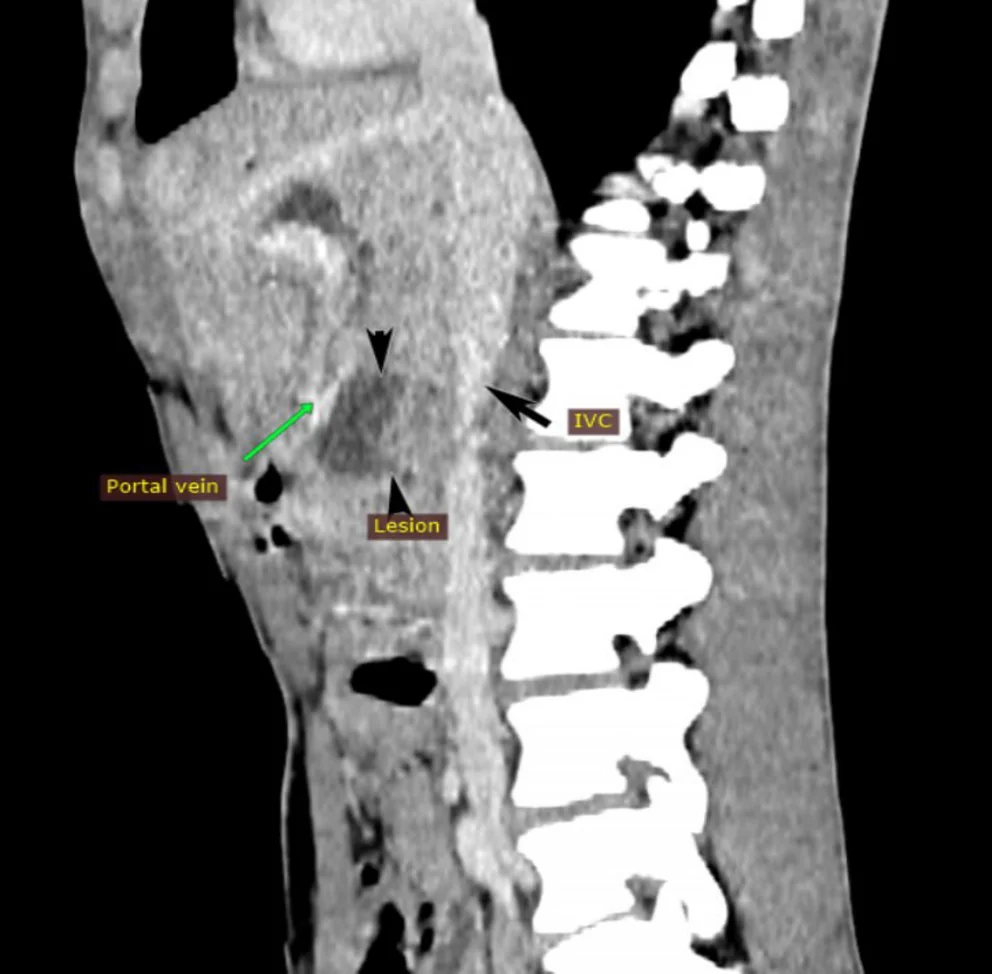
Sagittal contrast‐enhanced CT reconstruction through the porta hepatis demonstrating posterior compression of the inferior vena cava (IVC) and anterior displacement of the main portal vein by the periportal lesion.
